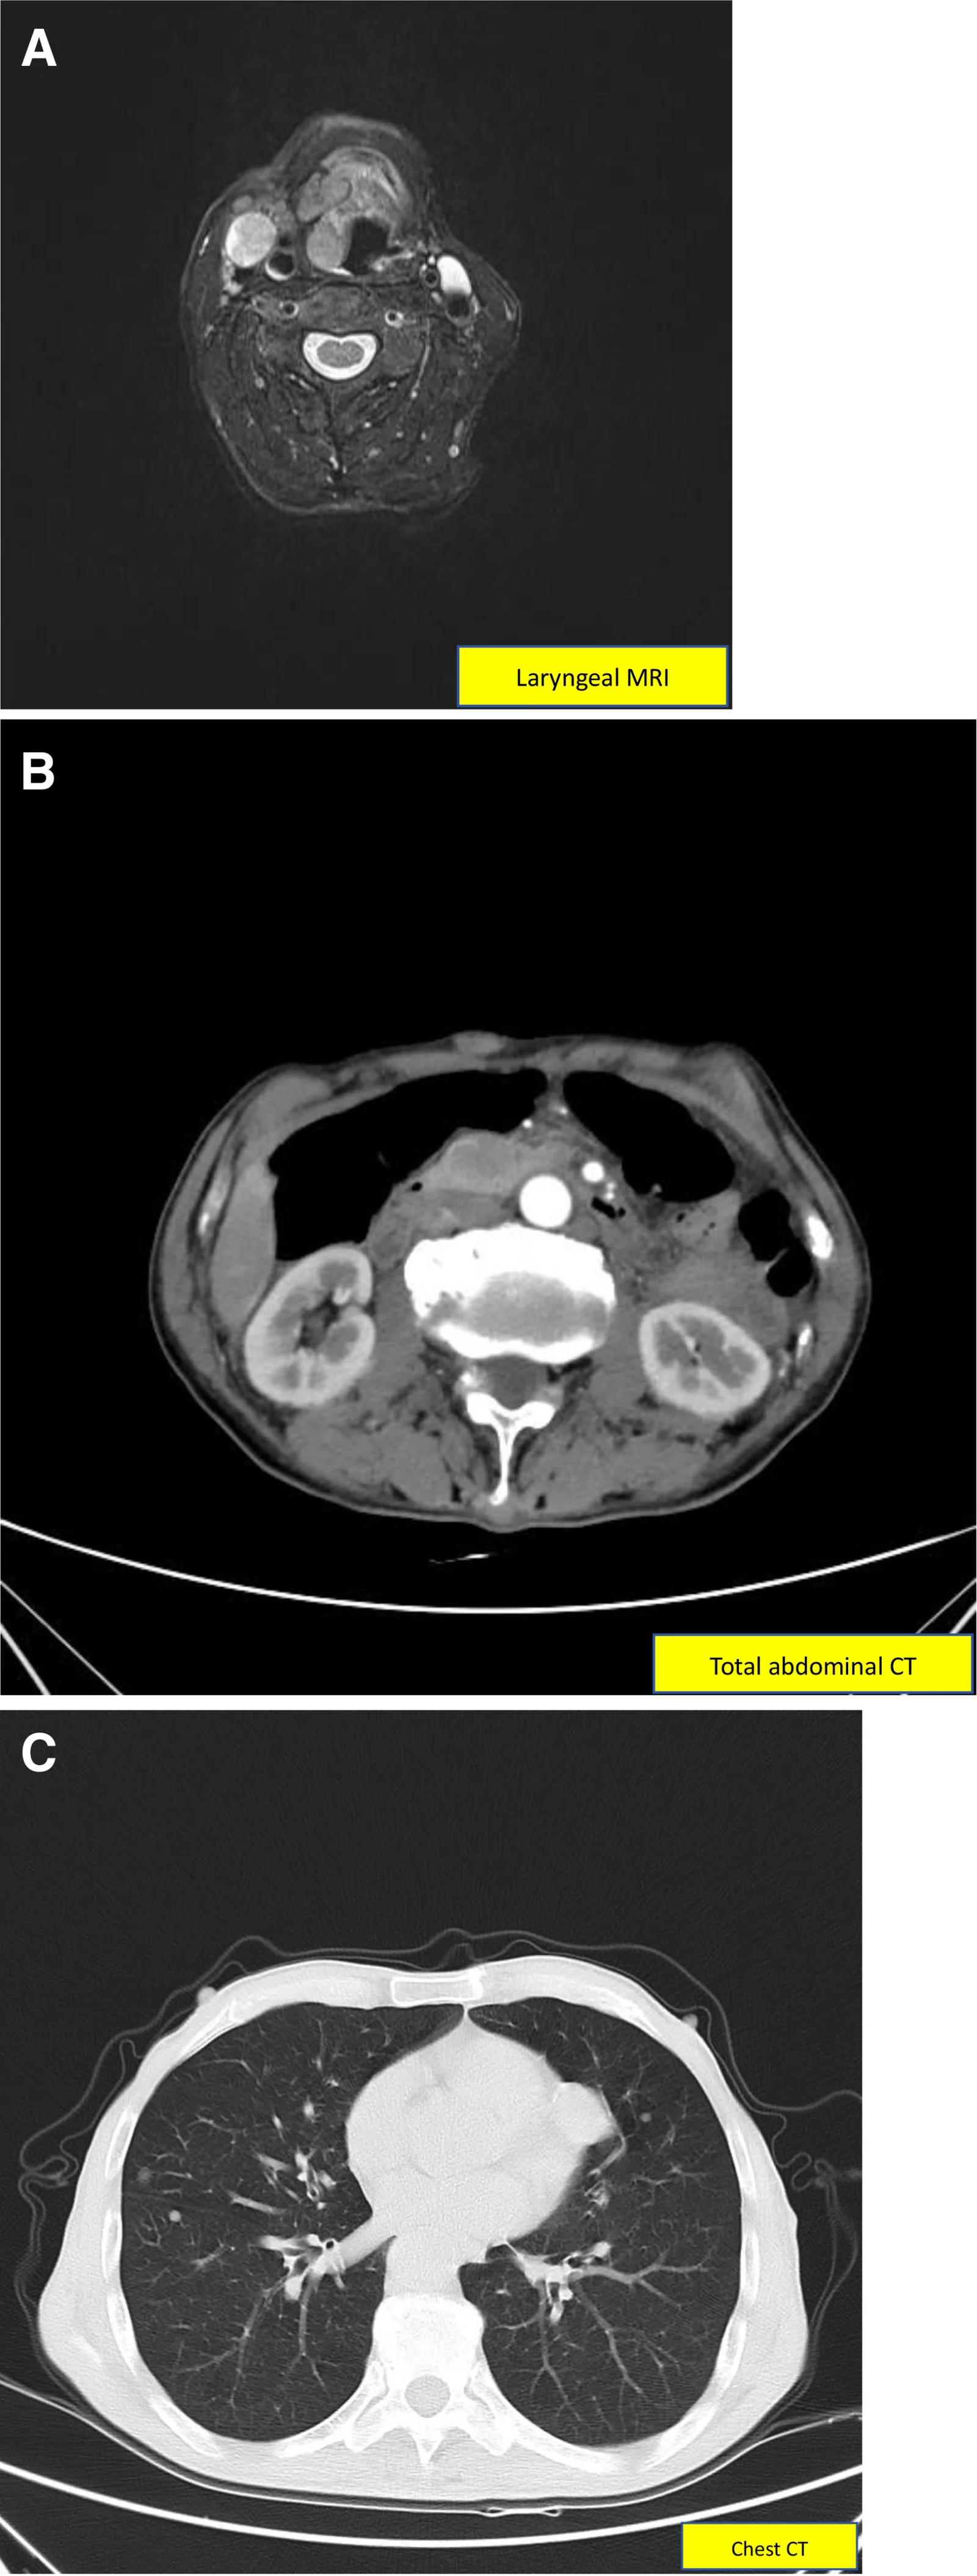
After partial laryngectomy: laryngeal MRI (T2WI) showed multiple slightly high signal nodules in the right supraglottic area and the right neck. Recurrence of paraganglioma with right cervical lymph node metastasis was considered (A). Total abdominal CT enhancement showed a pancreatic head occupying about 2.2 cm in diameter, with unclear borders, and metastasis was considered (B). Chest CT scan showed multiple metastatic tumors in both lungs (C). MRI = magnetic resonance imaging, T2WI = T2-weighted imaging.
